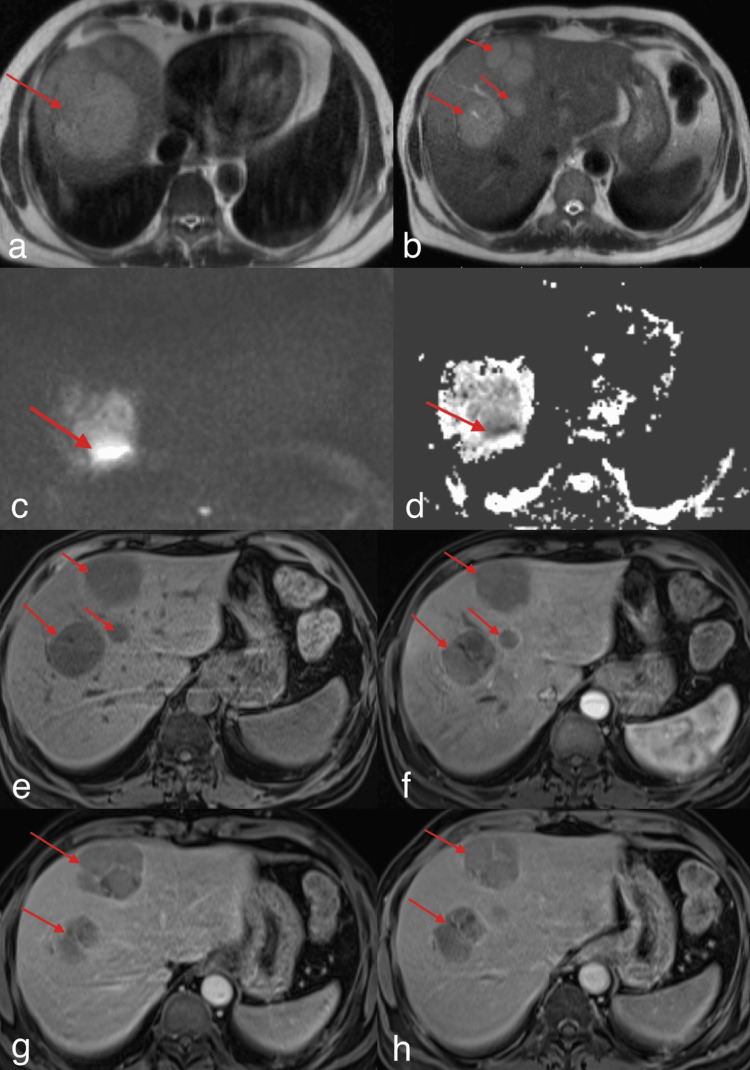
CE-MRI of the abdomen. Contrast-enhanced MRI (CE-MRI) showing lobulated T2 hyperintense lesions (a, b) showing peripheral diffusion restriction on diffusion-weighted imaging (DWI)/apparent diffusion coefficient (ADC) map (c, d) and T1 hypointense signal (e) with subtle peripheral and septal enhancement on post-contrast images in the arterial phase (f), venous phase (g), and delayed phase (h), indicated by red arrows.
